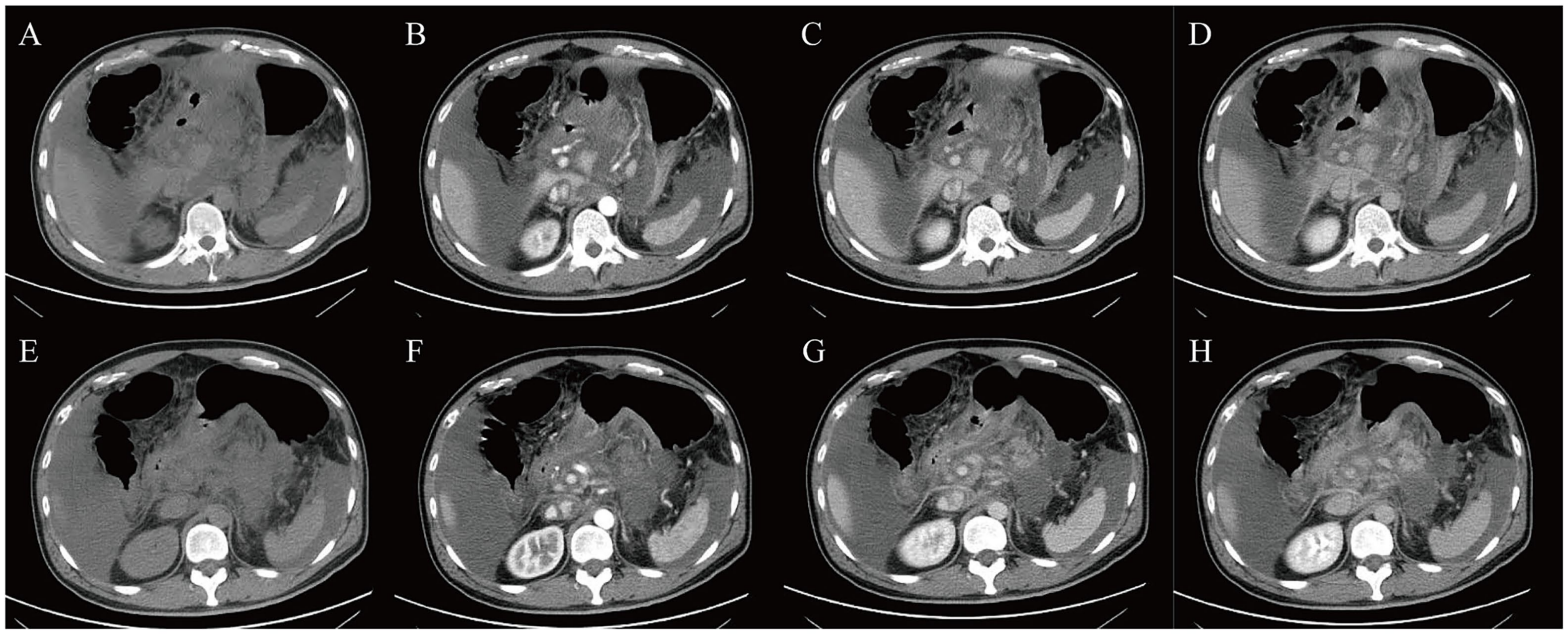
Contrast-enhanced CT scans. (A,E) plain CT, (B,F) enhanced CT arterial phase, (C,G) enhanced CT venous phase, (D,H) enhanced CT delayed phase.
